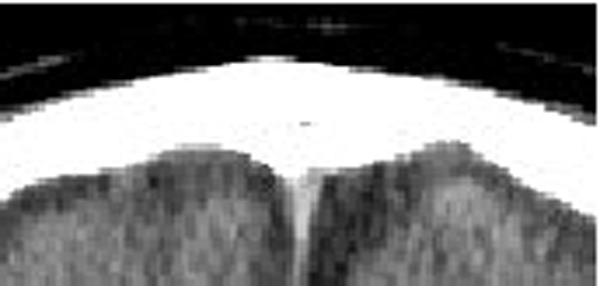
The anterior bone protrusion. This is the Region of Interest for bone protrusion detection. Most CT scans of the skull in this area show the protrusion feature.
